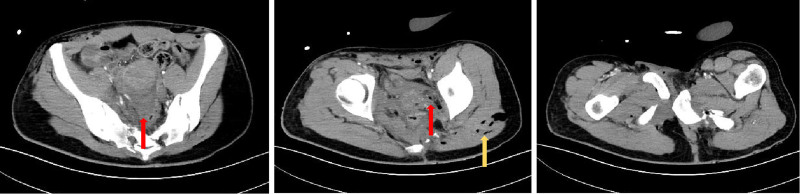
Computed tomography (CT) of the pelvis demonstrating an APC III type fracture, with fluid accumulation and free gas (red arrows), and extensive soft tissue damage (yellow arrows). APC = anterior-posterior compression .
